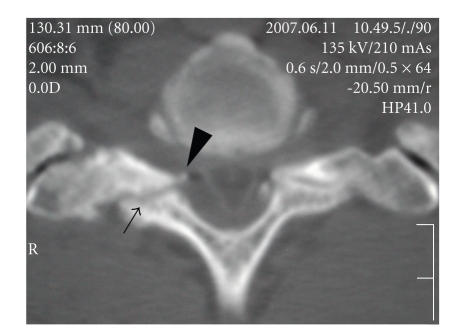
Computed tomographic myelography at C7/T1 level. The spinal cord is compressed from both sides and shows atrophy. The right C7/T1 facet joint indicates severe arthrosis (arrow) with bony spur extending into the spinal canal (arrowhead).
